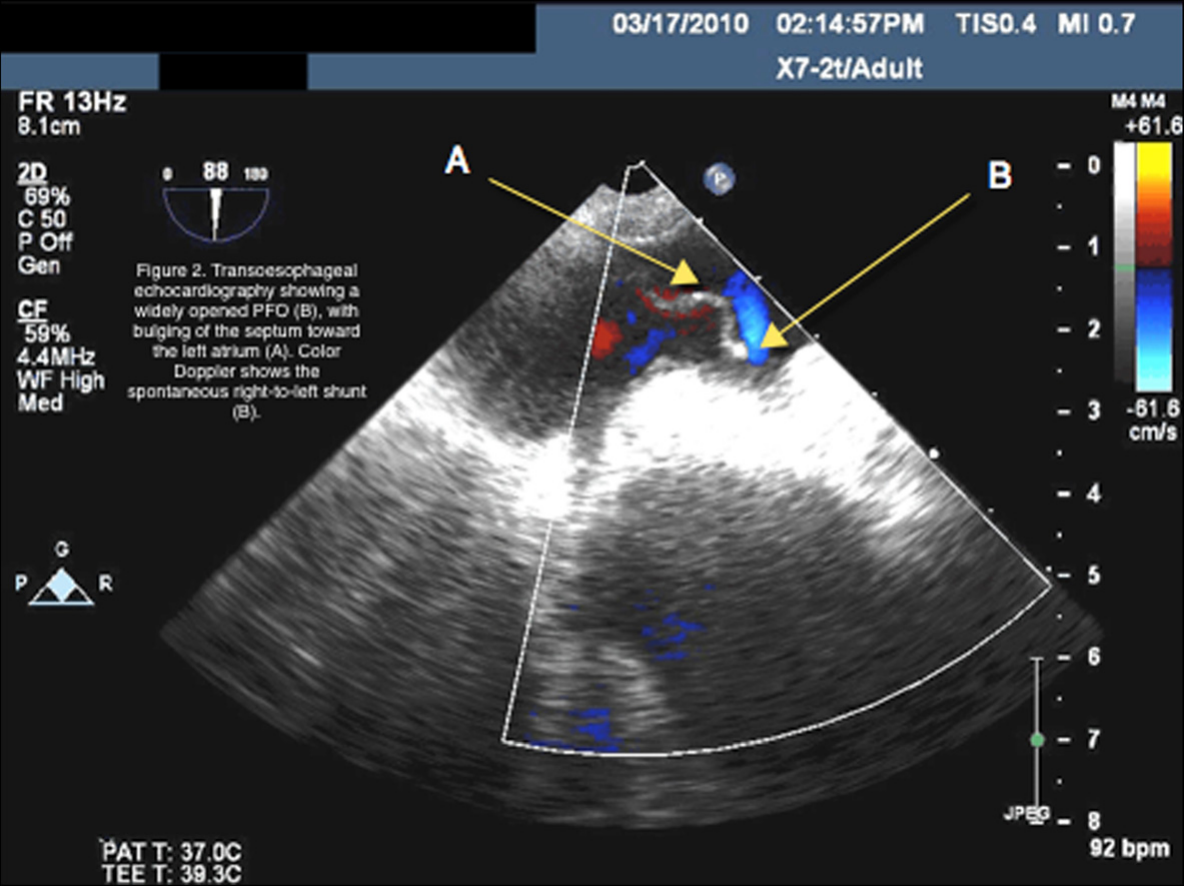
Post-Operative Transesophageal Echocardiogram reveals Widely opened PFO. Arrow A points to the bulging of the septum toward the left atrium. Arrow B points to the color Doppler showing the spontaneous right-to-left shunt
